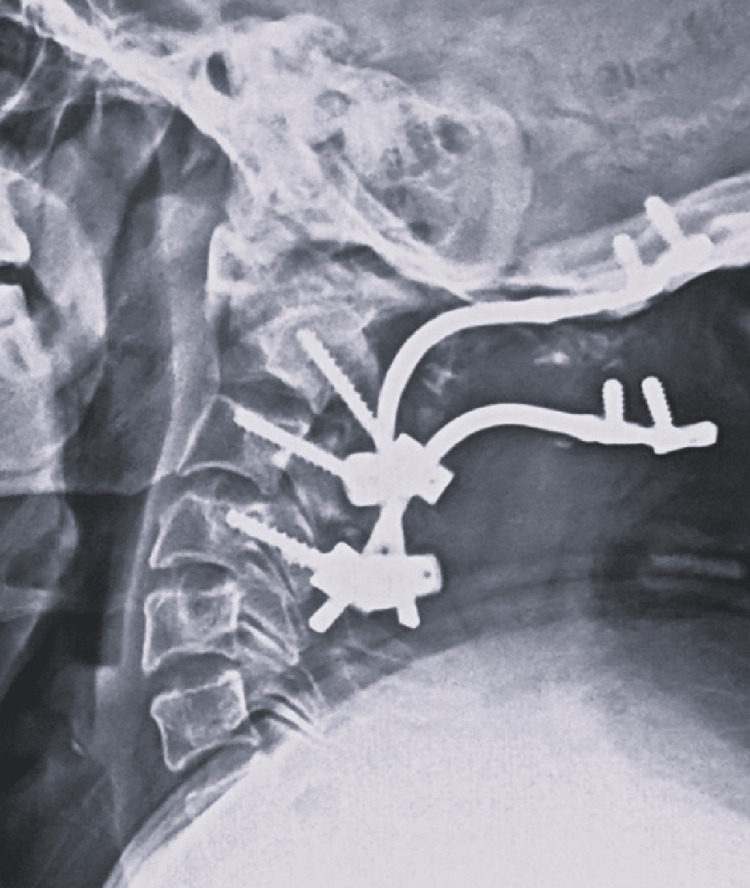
Lateral radiograph representing implant failure in a patient following OC fusion surgery for pathological odontoid fracture. OC fusion = Occipito-cervical fusion
